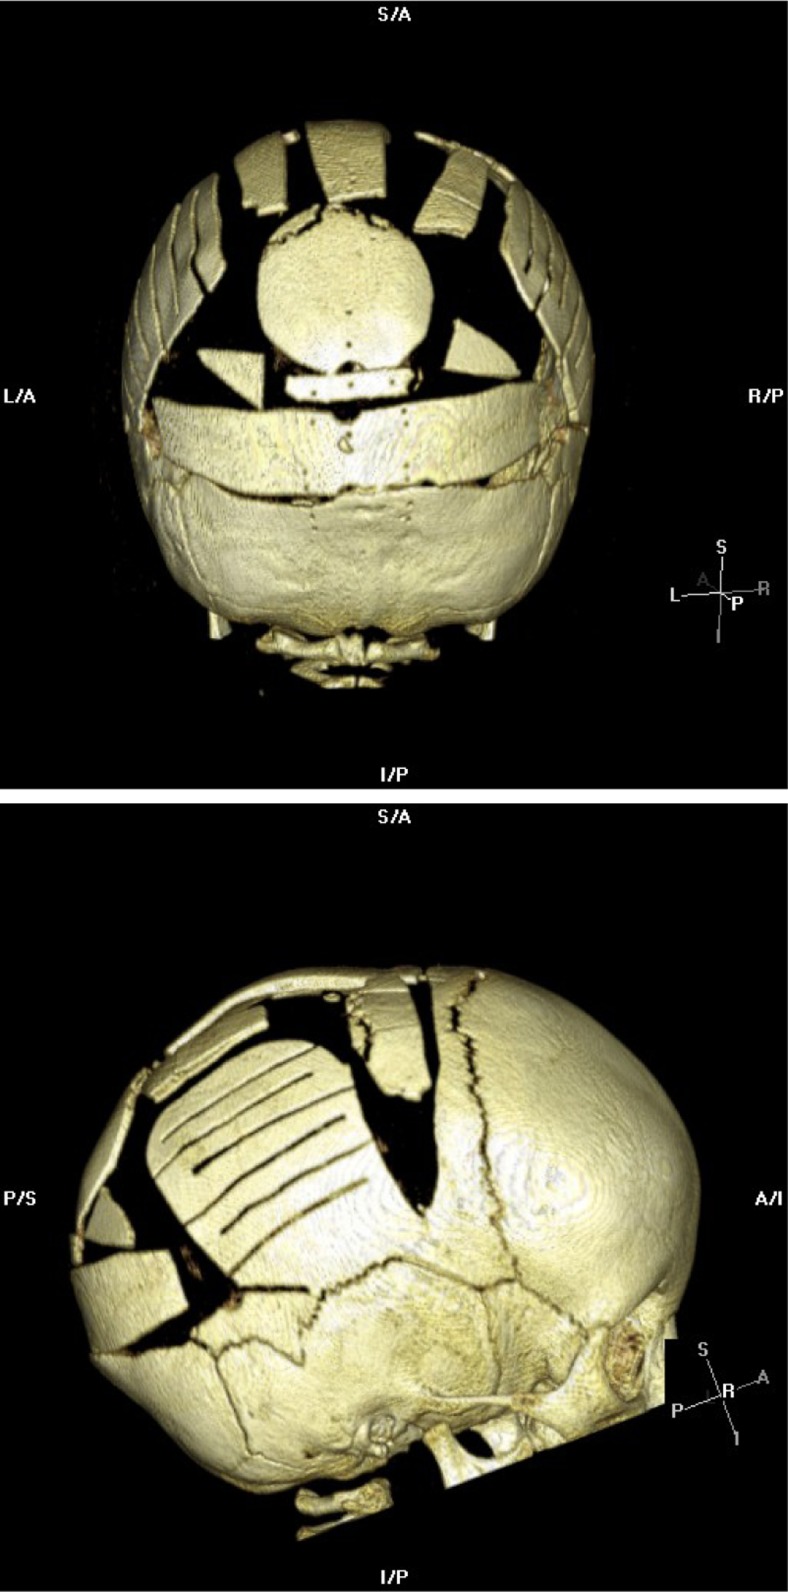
CT Multiplanar reconstruction (MPR) demonstrating the postoperative remodelled calvarium following craniosynostosis surgery
